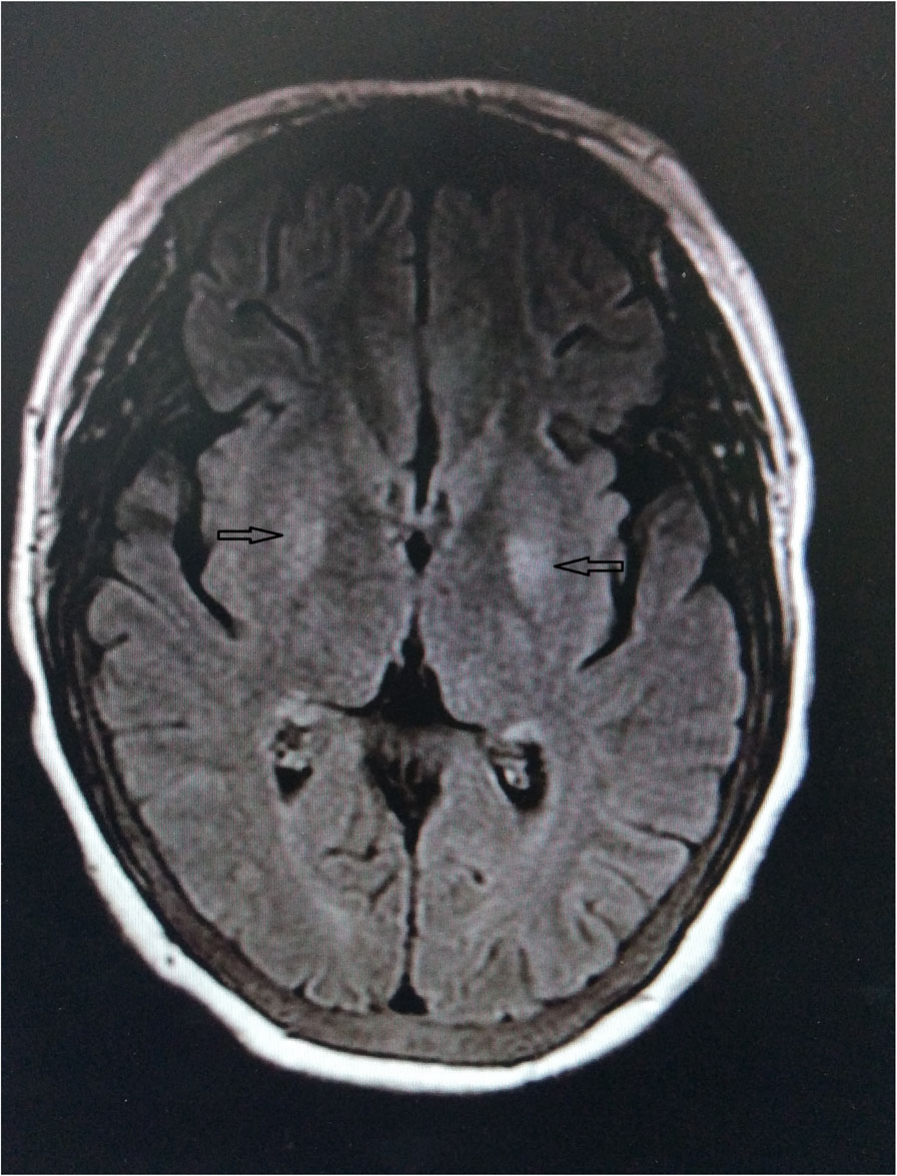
Follow-up MRI scan obtained after 14 days. T2FLAIR revealed high signal in the bilateral basal ganglia (arrows), which were significantly weaker compared to the initial MRI signal
